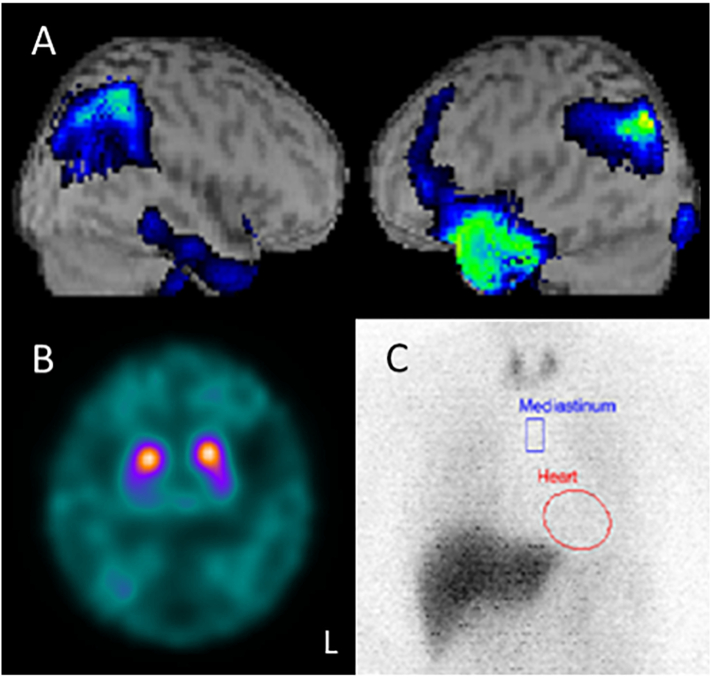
Nuclear imaging data. (A) ^123^I-isopropyl-iodoamphetamine single-photon emission computed tomography (^123^I-IMP SPECT) showed reduced cerebral blood flow in the left anterior temporal lobe and bilateral parietooccipital regions. (B) ^123^I-ioflupane single-photon emission computed tomography (DaT) scan showed reduced bilateral striatal uptake. (C) ^123^I-meta-iodobenzylguanidine (^123^I-MIBG) myocardial scintigraphy showed significantly reduced cardiac uptake.
